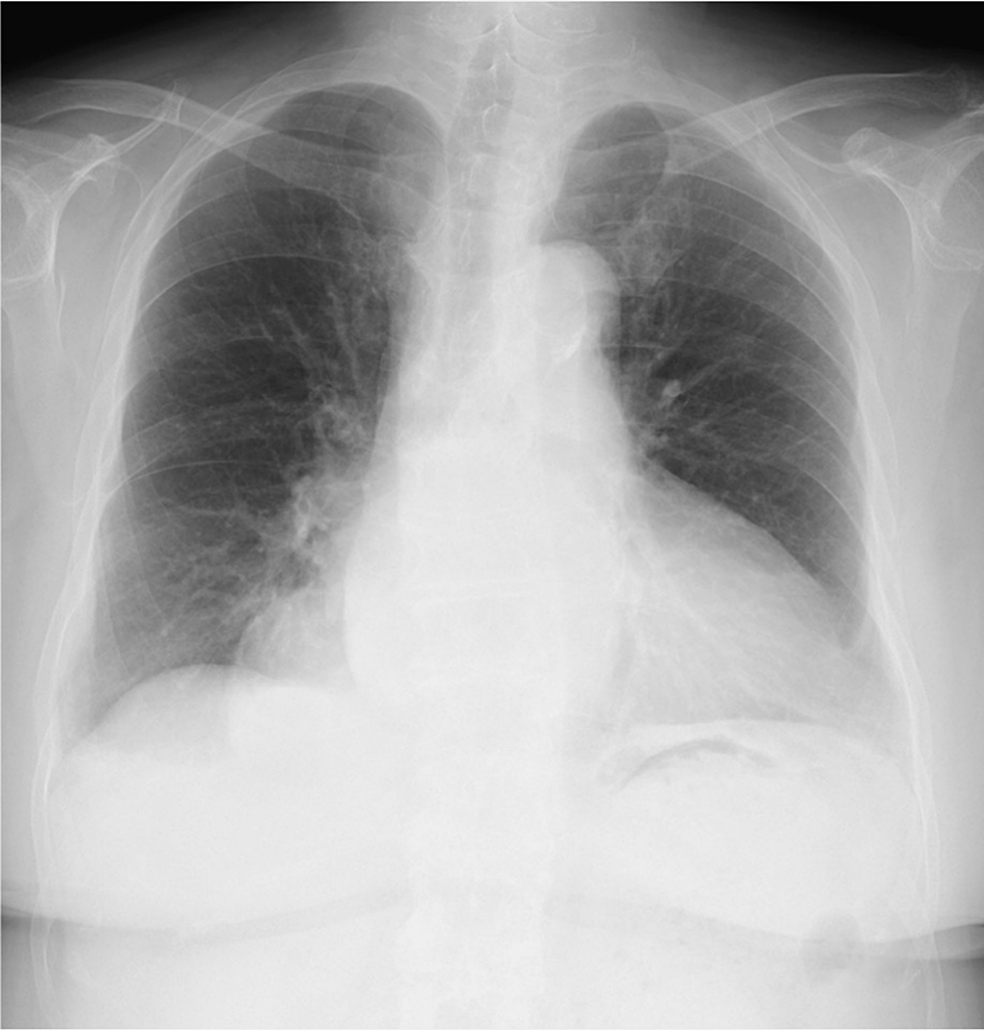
Chest radiograph (frontal view) An abnormal shadow is observed on the right lower lung field.
